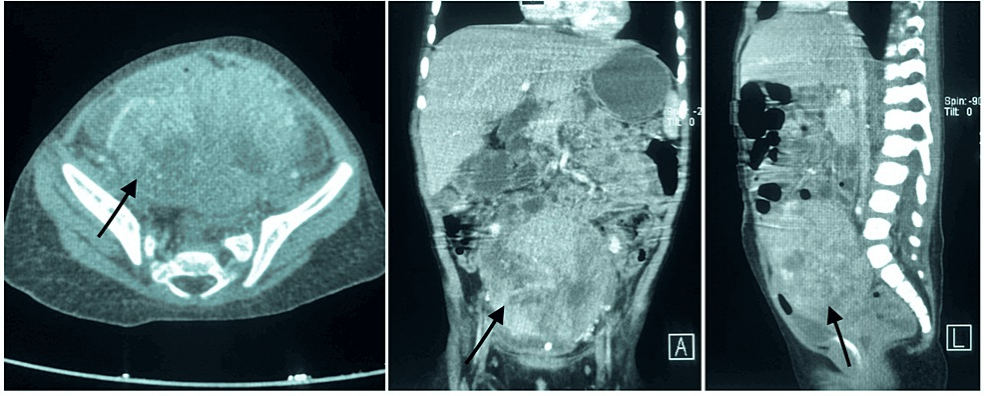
CT images at diagnosis. Dual-contrast CT scan of the abdomen and pelvis shows a heterogeneous mass with punctate calcifications, measuring 68x70x60 mm, centered in the uterus. The mass surrounds the left external iliac artery approximately 180° and the internal iliac artery 90°, without any cleavage planes. The scan also reveals a displaced and collapsed bladder. No lesions suggestive of liver metastasis were observed (see arrows).
